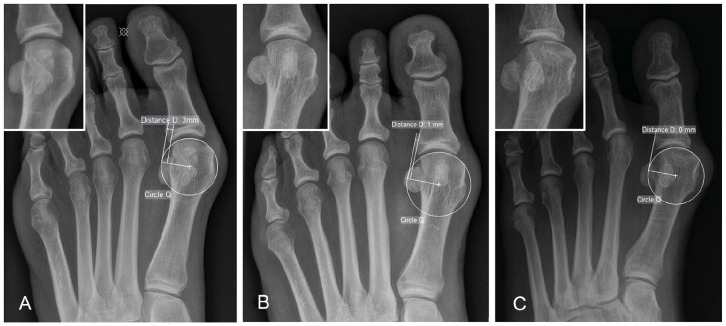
Dorsoplantar weightbearing foot radiographs with assessment of the round
